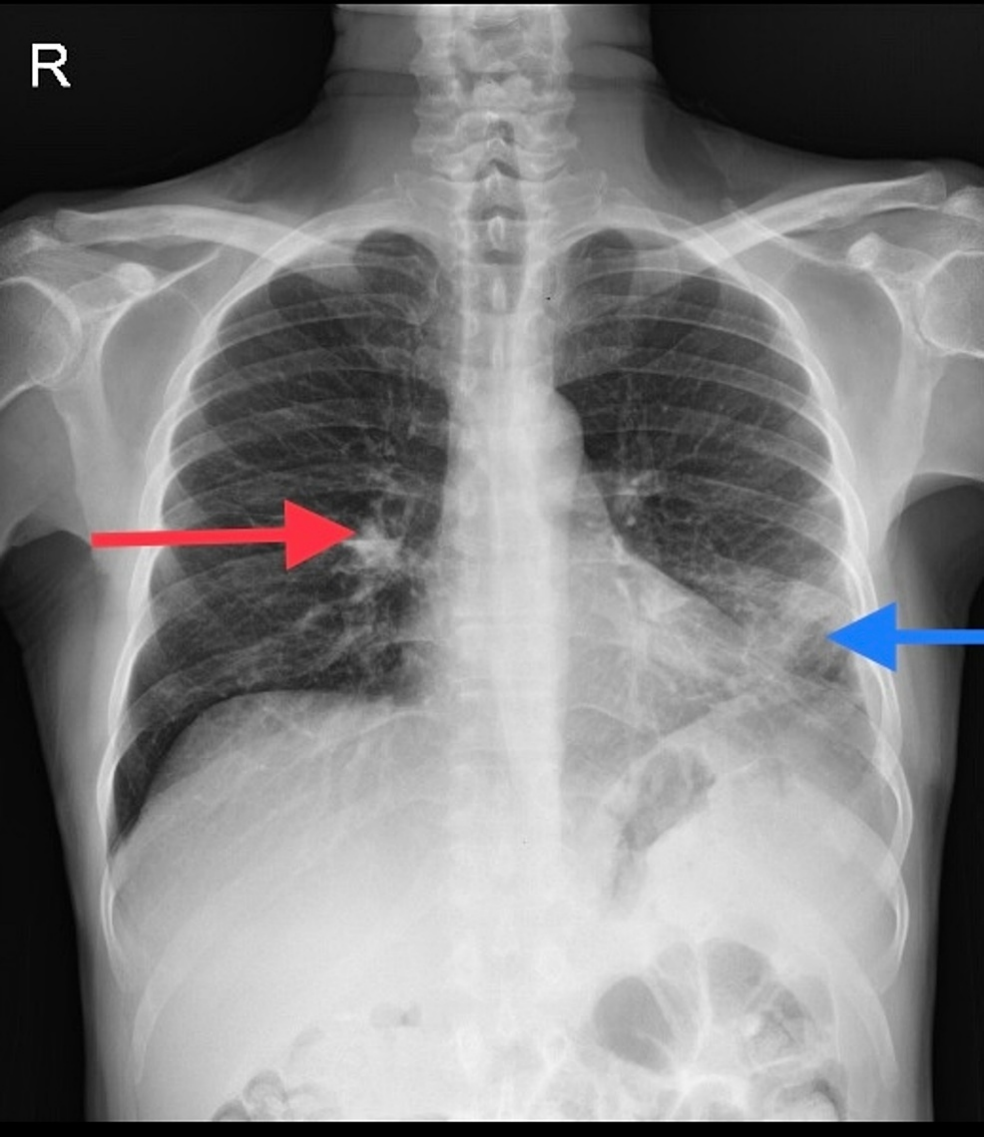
Chest X-ray showing right middle lobe (red arrow) and left lingular consolidation (blue arrow).
